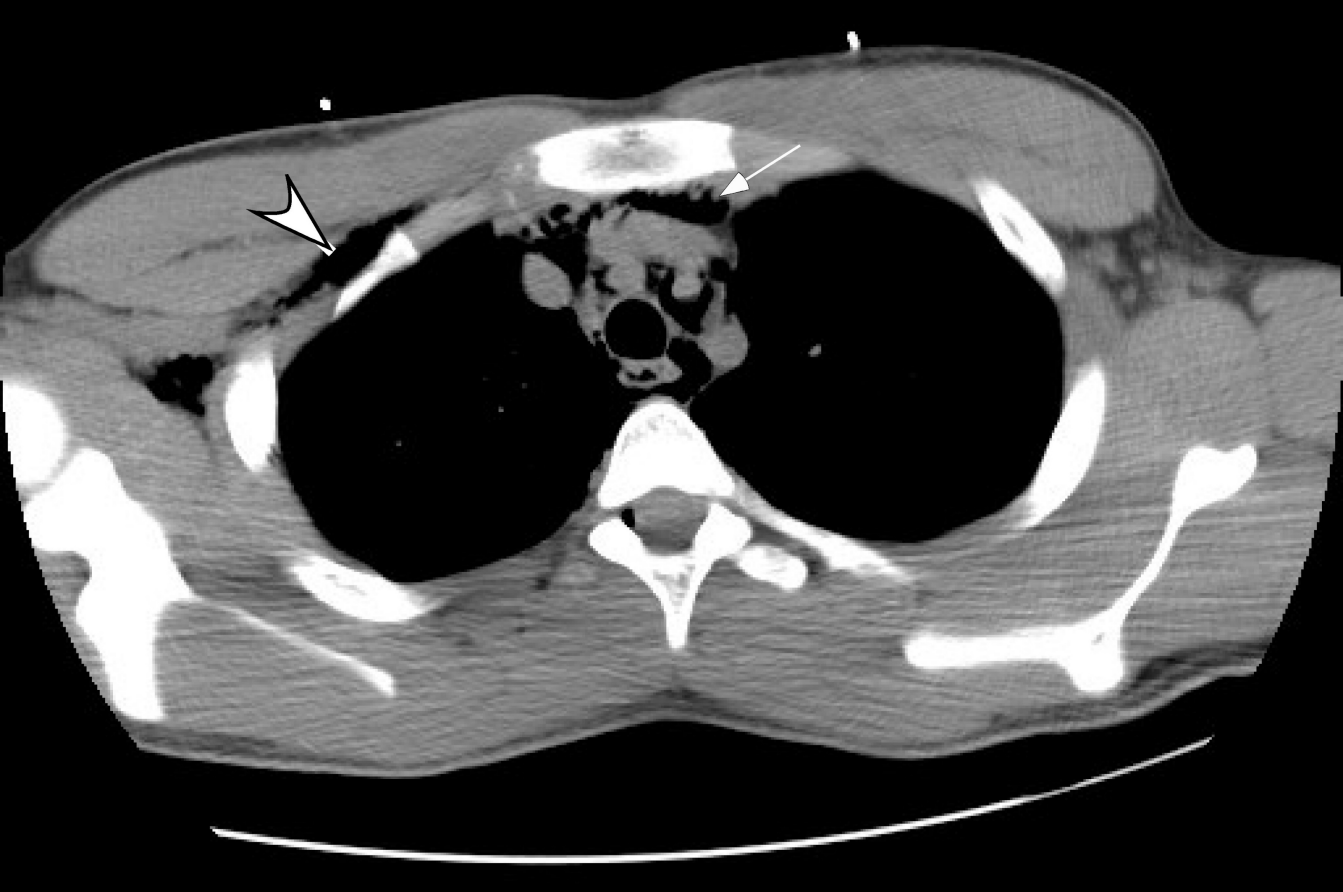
The computed tomography (CT) confirming the subcutaneous emphysema (arrowhead) and the extensive pneumomediastinum (arrow).
